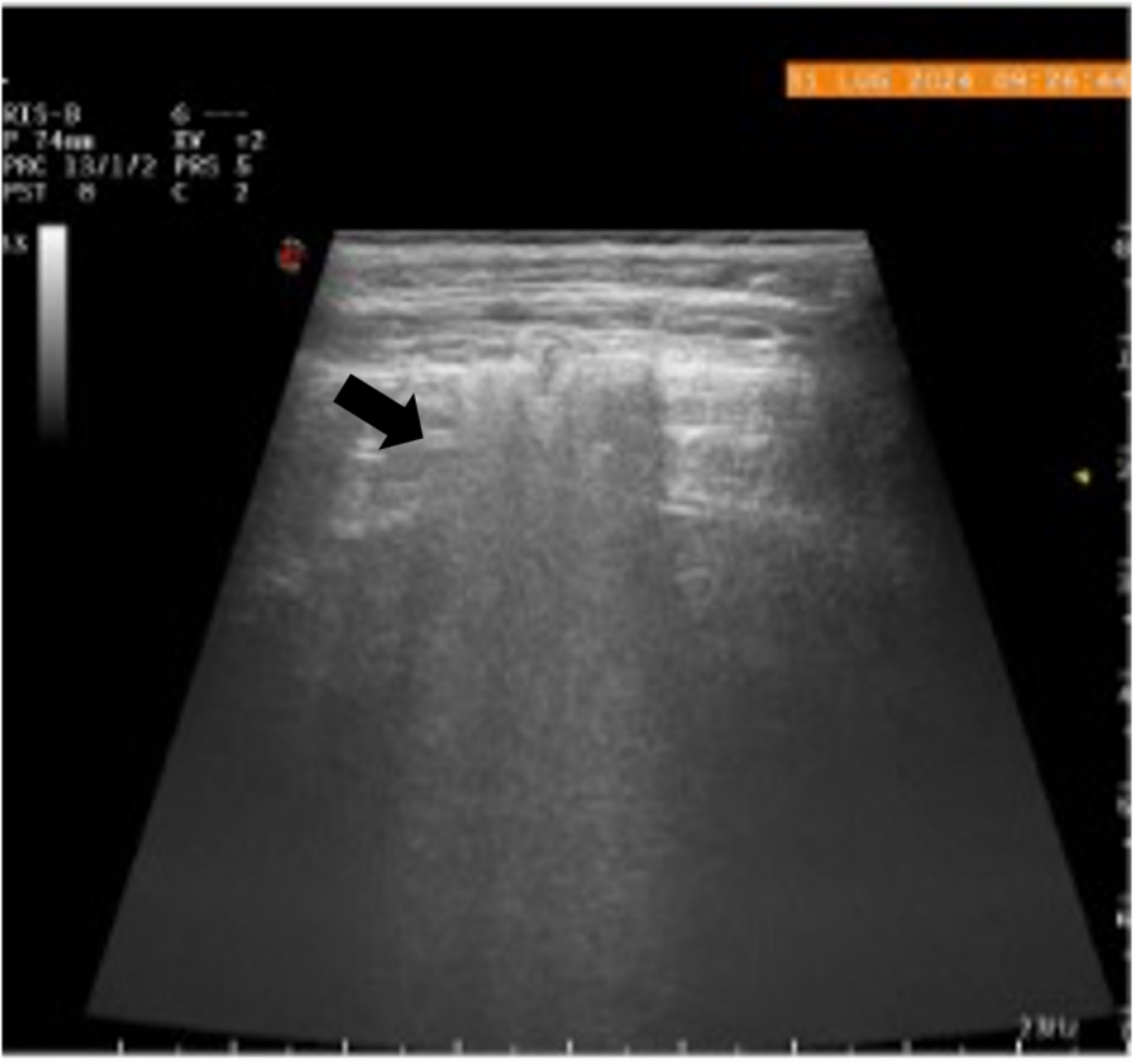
Ultrasound examination revealed a subpleural hypoechoic area surrounded by an interstitial pattern, consistent with pneumonia
